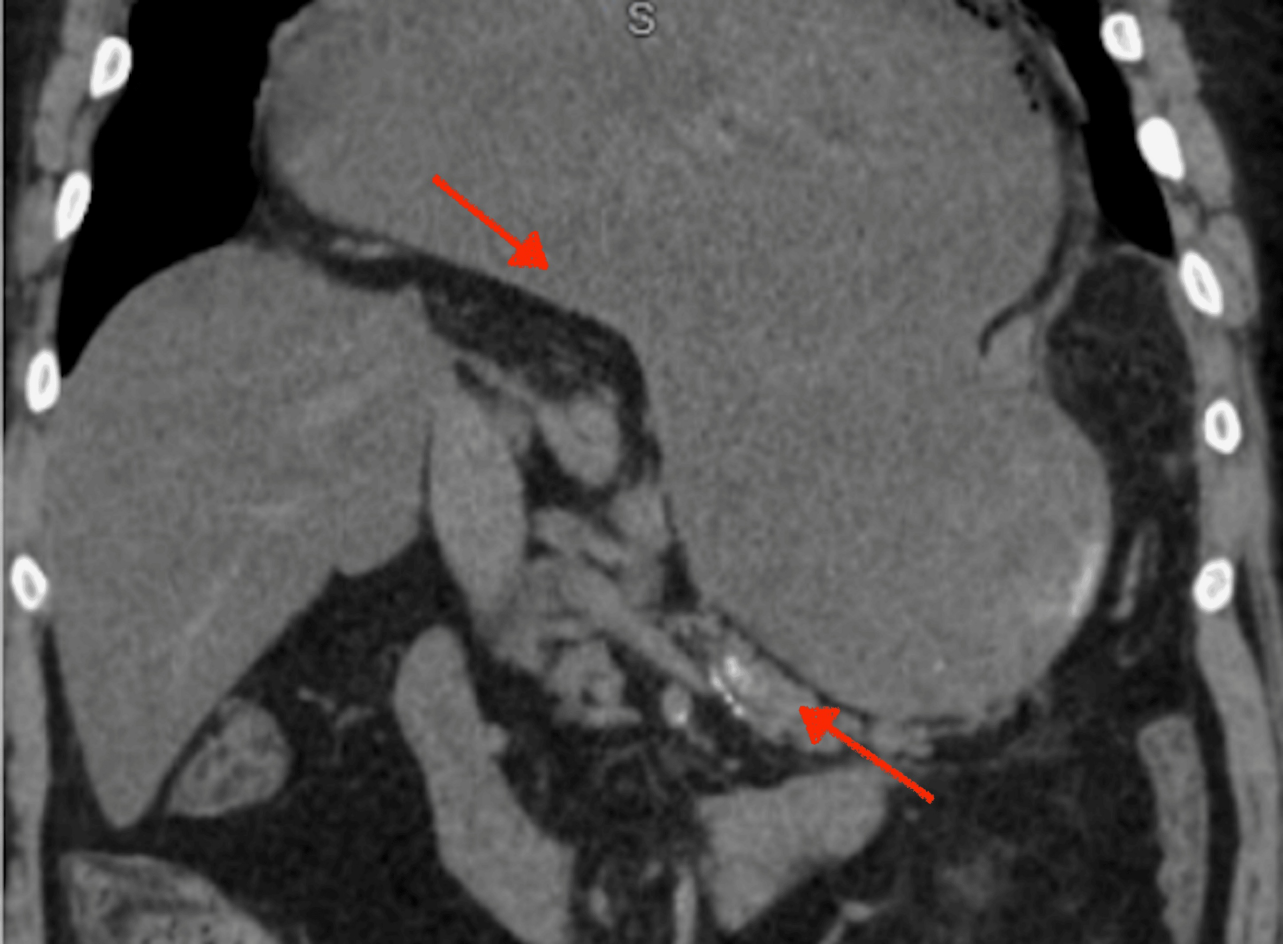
Coronal computed tomography demonstrating intrathoracic displacement of the stomach with a transition point reflecting abnormal rotation (arrows) These findings are consistent with mesenteroaxial gastric volvulus in the setting of a large hiatal hernia.
